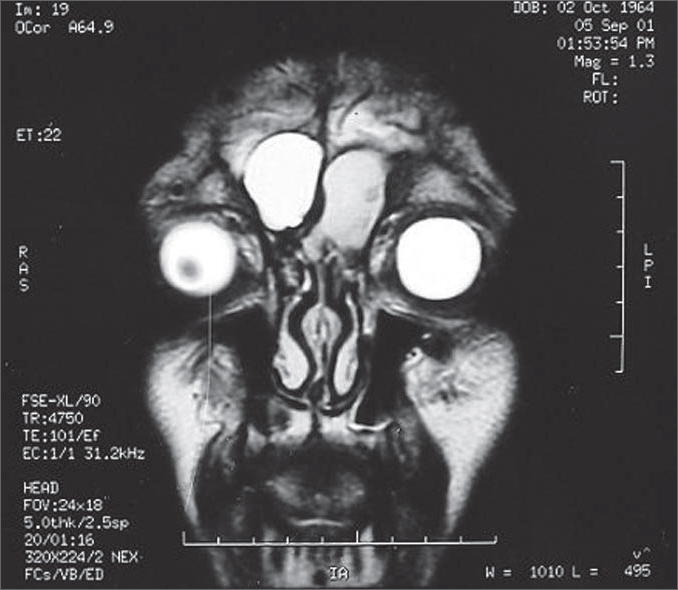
Magnetic resonance image of paranasal sinuses, coronal section, T2. Bilateral Frontal Mucocele.
